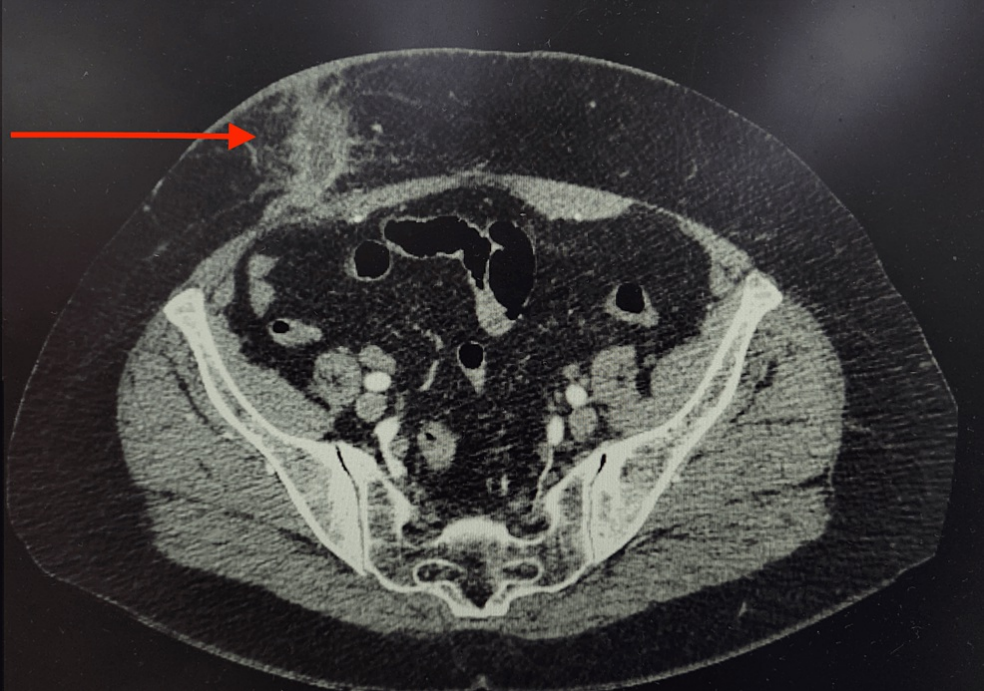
CT abdomen: an area of subcutaneous fat densification and liquefaction with the fistulous tract.
